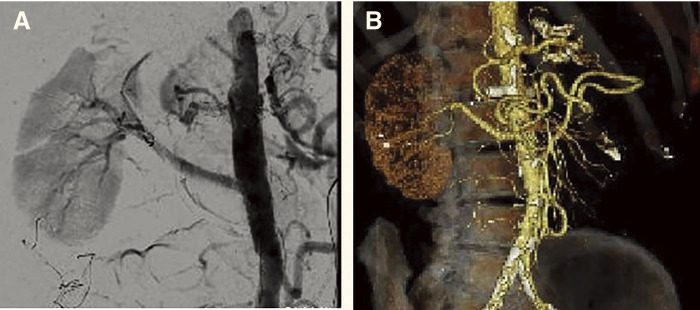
The aorto-renal bypass was patent on intraoperative angiography (A), and the right renal artery was well-contrasted in the peripheral region on postoperative CTA (B). CTA: computed tomography angiography
